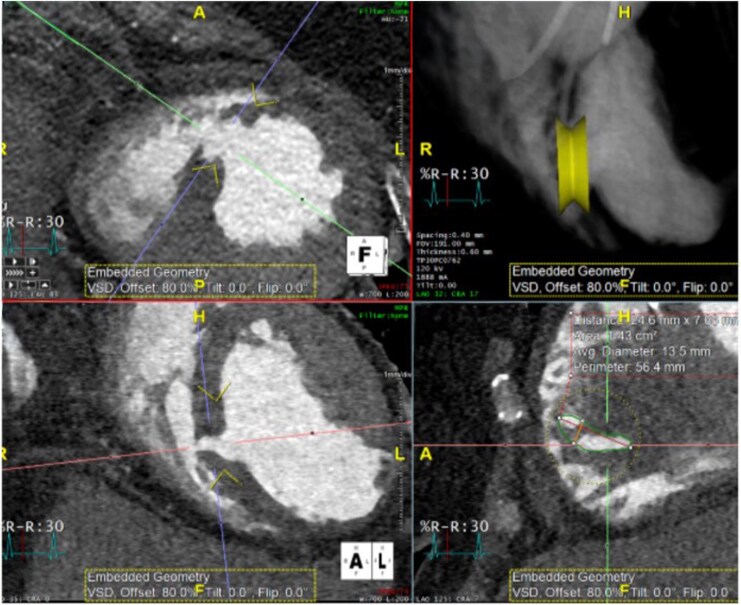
Preprocedure cardiac computed tomography displaying a 4 mm apical VSR and 3D rendering of planned VSR closure device.
